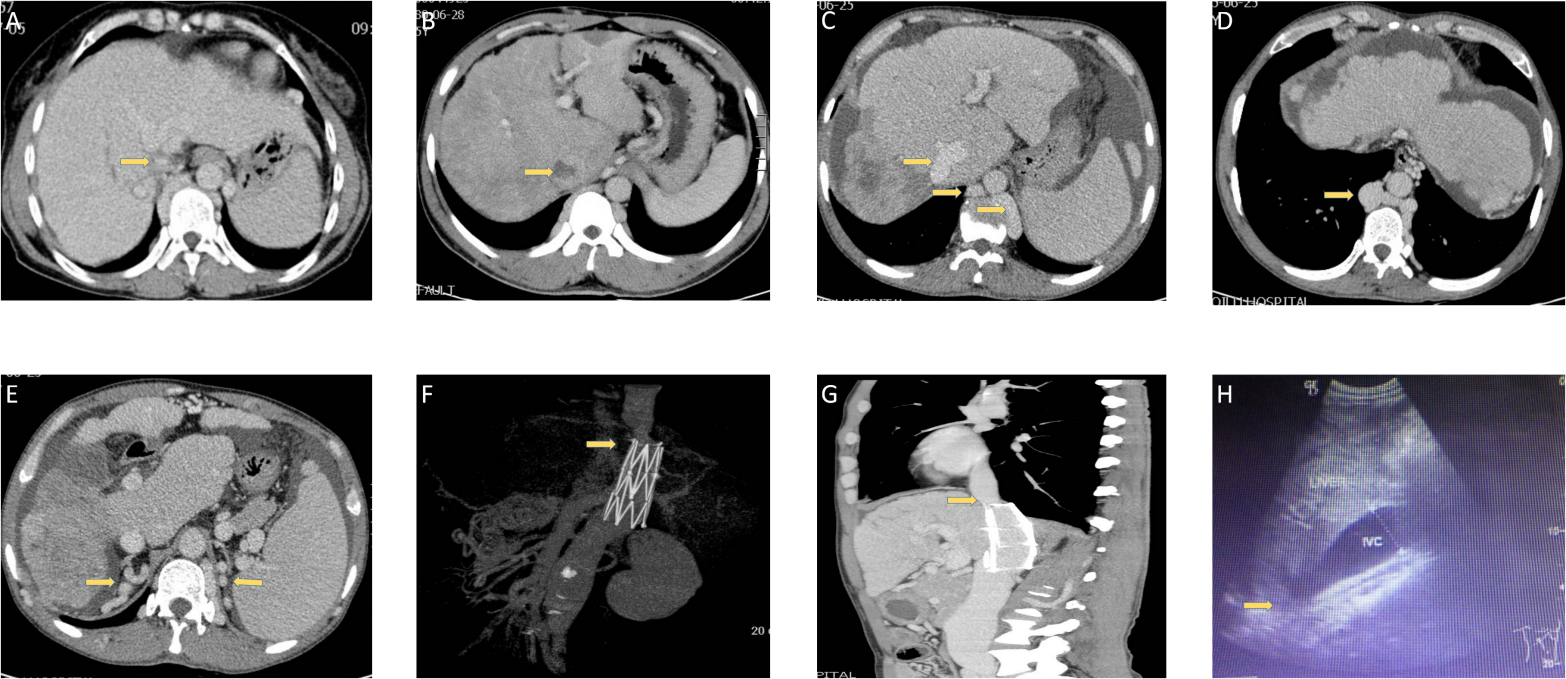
Different kinds of obstructions in BCS patients are complicated with HCC. (A) The narrowness of IVC in one patient. (B) Thrombosis in one patient. (C, D) IVC vascular malformation in one patient. (E) Compensatory vascular varicosity in one patient with no signs and symptoms. (F, G) CT angiography and a coronal section showing the segmental obstruction of IVC and corrected by stenting in one patient. (H) Ultrasonic imaging in one patient.
